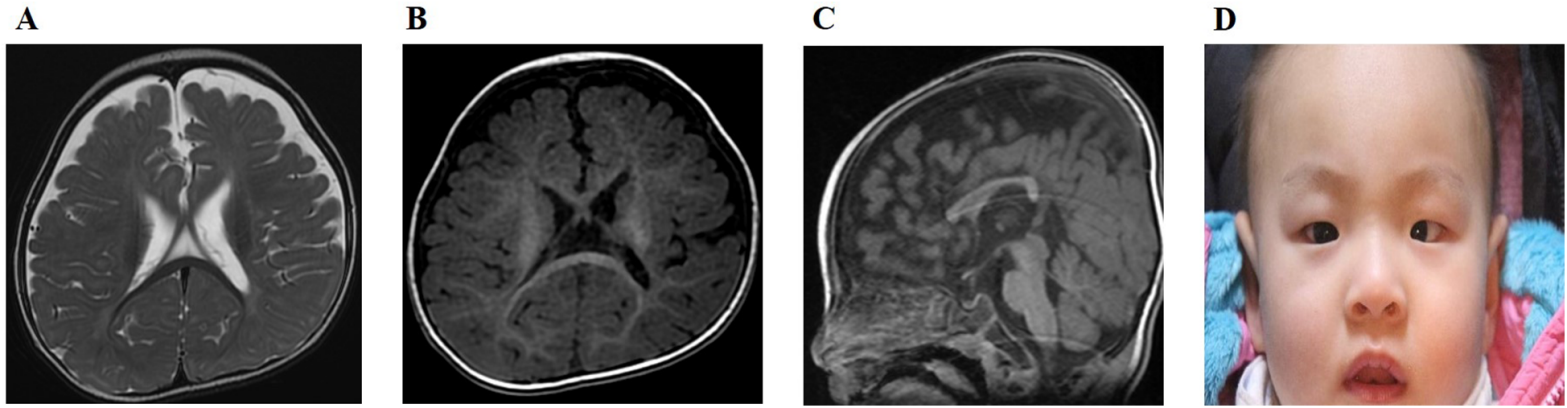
(A) Axial T2-weighted image of the proband at 10 months shows marked brain atrophy, particularly in the white matter. (B) Axial T1-weighted image of the proband at 10 months displays delayed myelination. (C) Sagittal T1-weighted image of the proband at 10 months demonstrates thinning of the corpus callosum. (D) At the follow-up examination at two years of age, the proband exhibited mild facial dysmorphism characterized by downward slanting palpebral fissures, hypertelorism, esotropia, and a tented upper lip vermilion.
